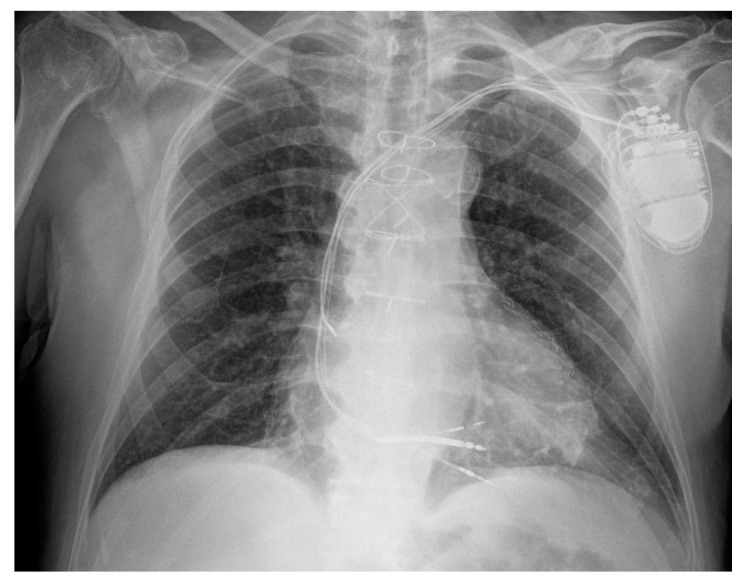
Post-procedural chest X-ray showing the implanted device, the connected leads (atrial lead, 3830 lead for LBBAP, right ventricular defibrillator lead), and the abandoned right ventricular pacing lead.
